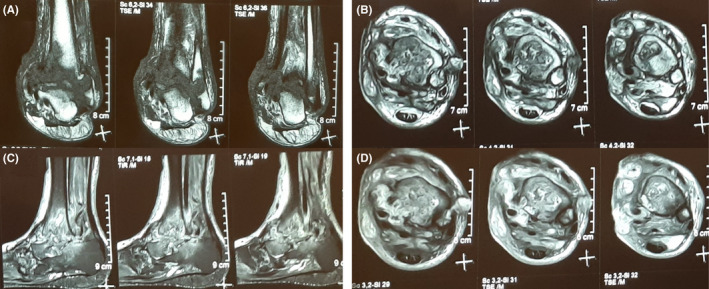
(A) coronal T1‐weighted, (B) axial T2‐weighted, (C) sagittal Short Tau inversion recovery (STIR), and (D) diffusion‐weighted (DW) magnetic resonance images (MRI) of ankle and foot of the patient. Severe cartilage loss and bone destruction in the ankle joint are evident. Also, bone marrow edema and intermediate to low‐signal lesions in the tibia and fibula with loculation in subcutaneous and extension to the skin can be observed. Moreover, the lesions are high signal in DW images
